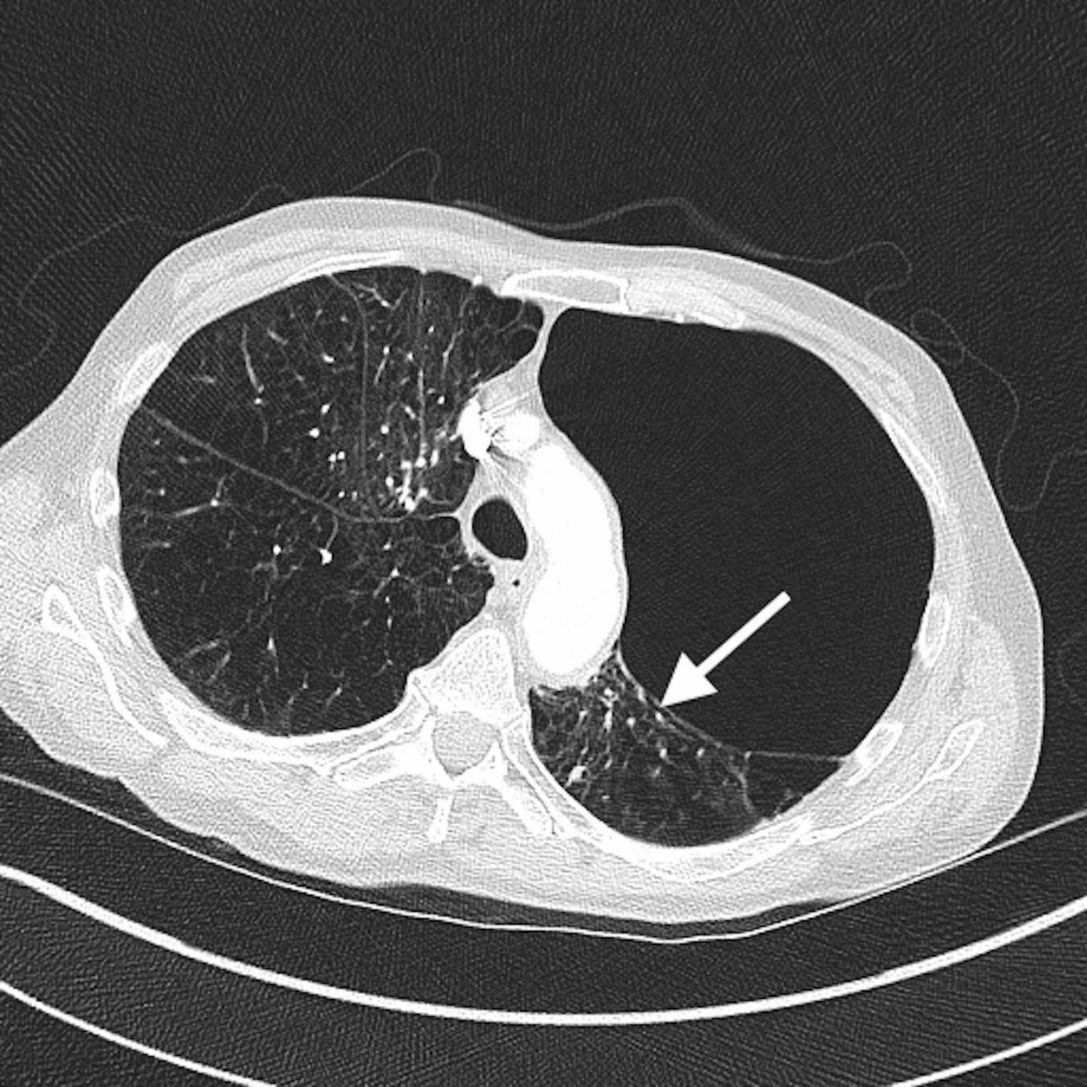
CT scan of the chest Axial section of CT showing severe bilateral emphysematous changes with a giant bulla involving left upper lobe, compressing left lower lobe parenchyma (arrow).
